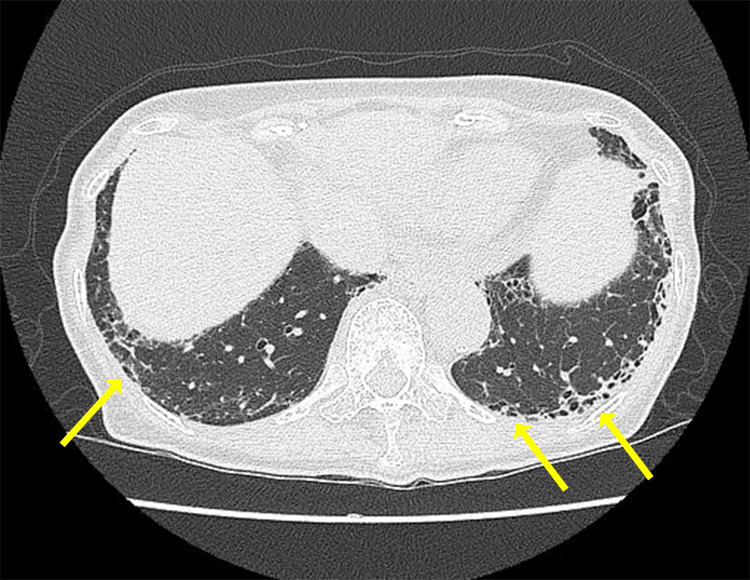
Computed tomography (CT) of the chest Reticular opacities are observed in the peripheral lung bases, consistent with mild interstitial pneumonia.
